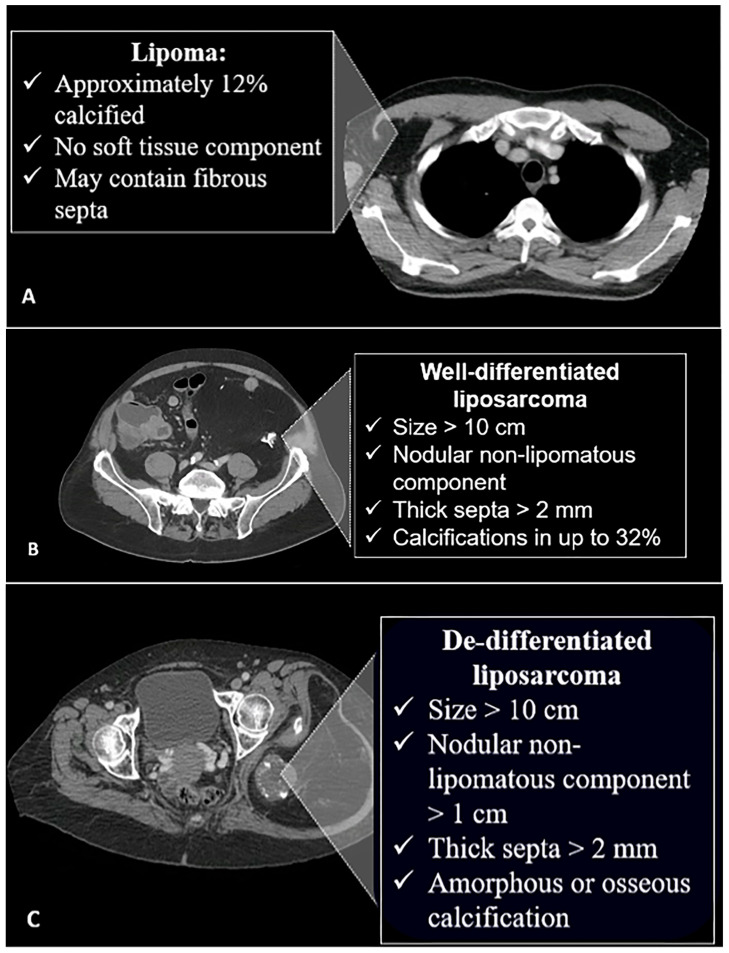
Characteristic features of different fat-containing lesions on CT scan. (A) Lipomas may contain septa and might have calcifications in 12% of cases. (B) Well-differentiated liposarcomas are larger, with non-lipomatous nodular components and thickened septa. Calcifications are more common and seen in 32% of cases. (C) In the de-differentiated form, the lesions are larger and may contain amorphous or osseous calcifications. The image was obtained from the University of Washington Medical Imaging database with the required permissions.
